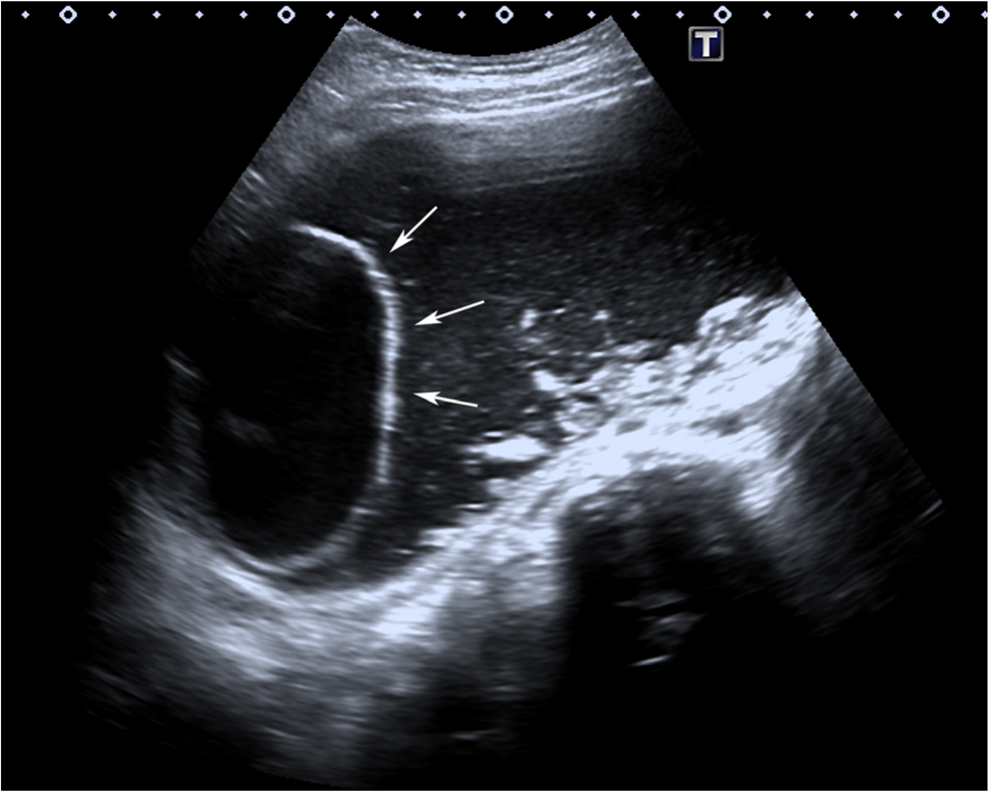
Transverse sonogram of a 14-year-old female with mature cystic teratoma demonstrates a cystic Rokitansky nodule (arrows) in a cystic mass. Note that there is echogenic debris at the gravity-dependent portion of the cyst
